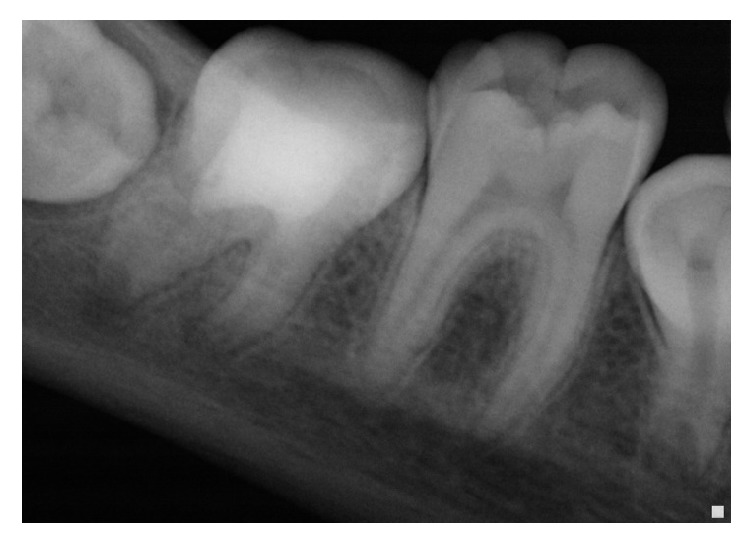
Radiograph after 3 months' recall: reduction of the radiolucent image at the distal root.
